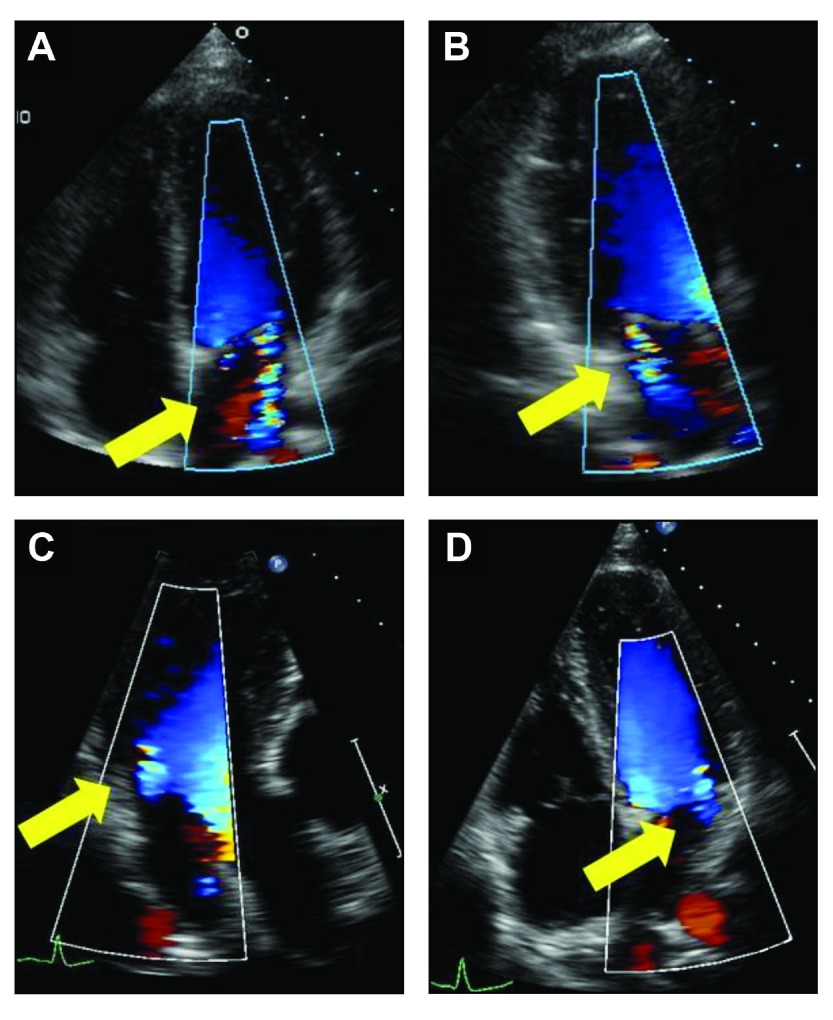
Echocardiograms: baseline (
